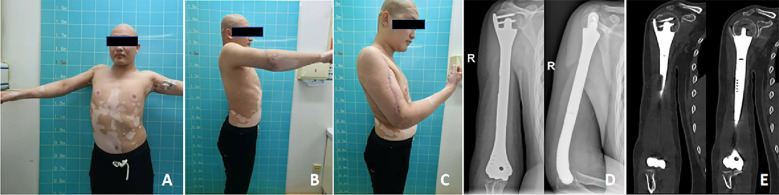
Nine months postoperatively, the patient’s shoulder and elbow joint function was assessed using imaging. (A–C) The patient’s right shoulder joint demonstrated active abduction to 75°, forward flexion to 80°, while the elbow joint exhibited a passive range of motion from 0°to 90°. (D) Anteroposterior and lateral radiographs of the right humerus showed proper prosthesis positioning, well-integrated bone-prosthesis interfaces, and good elbow alignment. (E) An artifact-free CT scan of the right humerus revealed successful integration at the 3D-printed prosthesis-bone interface, with appropriate alignment of the right elbow joint.
